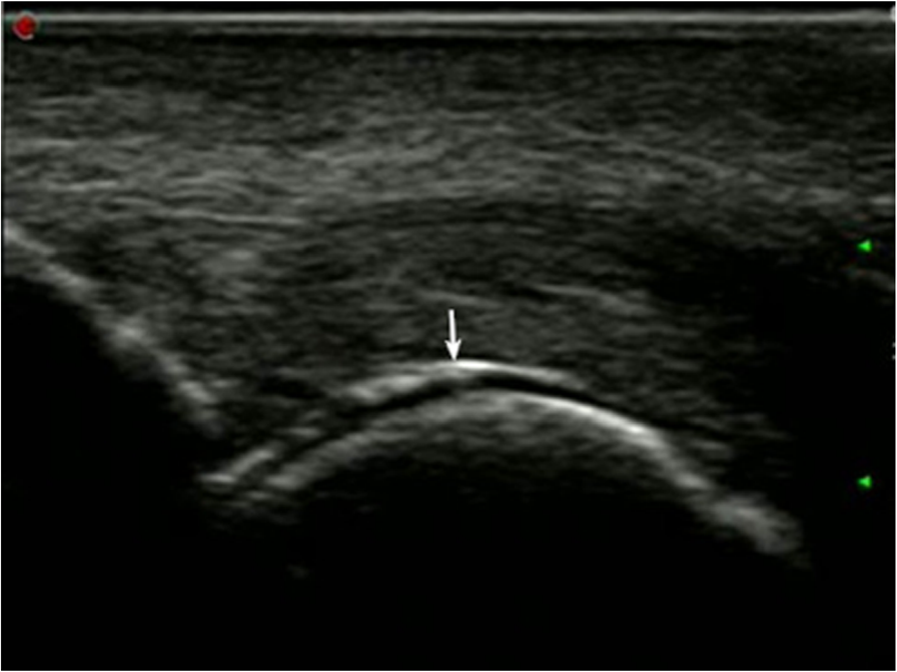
The ultrasound image for double-contour sign in gout patients. The white arrow represented the signal of double-contour sign in gout patients
